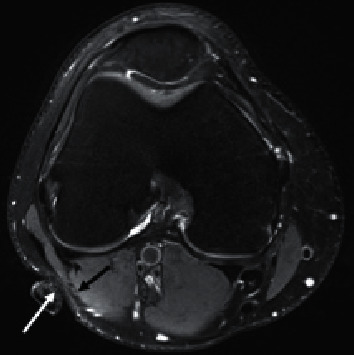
URIS^®^ active electrode position on the skin in the popliteal fossa and the peroneal nerve course. The magnetic resonance (MR) scan demonstrating the URIS^®^ active electrode (for the MR imaging purposes, the electrode was replaced by a wet cotton swab) marked with a white arrow located in the immediate vicinity of the peroneal nerve (marked with the black arrow).
